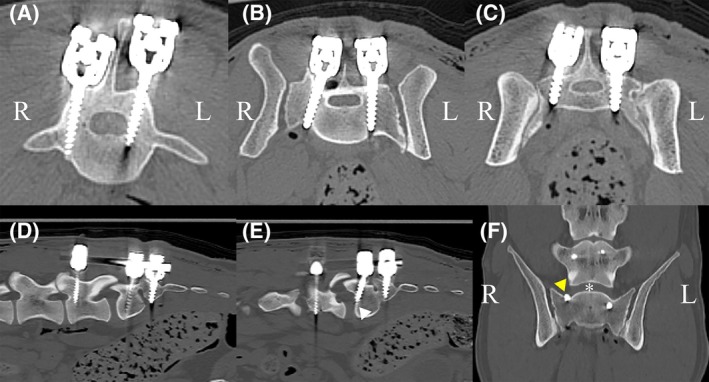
Postoperative computed tomography (CT) images of the clinical case. Postoperative CT images of the clinical case demonstrating pedicle screw positions at L7, S1, and S2. Transverse views (A–C), sagittal views (D, left; E, right), and a dorsal view (F) are shown. (A) The L7–left pedicle screw exhibits a grade 1 medial canal breach. (E) The S1 pedicle screw traverses the cranial endplate (white arrowhead), indicating a cranial breach grade 2. (F) There is no intradiscal penetration; the disc is marked by an asterisk, and a mild scuffing injury is visible along the lateral aspect of the disc (yellow arrowhead). L, left; R, right.
